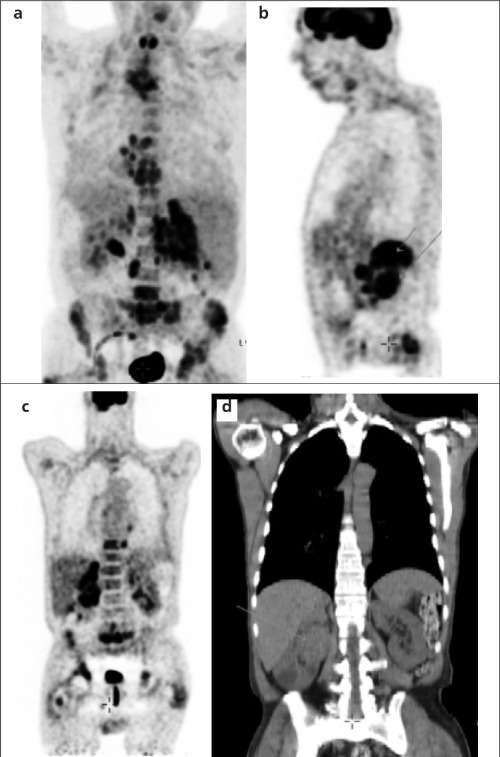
F-18 FDG PET/CT images showing thoracic and abdominalmultiple para-aortic hypermetabolic lymph nodes, increased F-18 FDGuptake in multiple foci of thoracic and lumbar vertebrae, and in thepelvic bones with osteolytic lesions showing up as patches on CT(a: MIP image; b: sagittal section; c: coronal section and d: coronal CTsection). There was clearly a pathologic uptake of the soft tissuelesion originating from the right kidney measuring 6x5x3 cm andbilaterally in the surrenal glands (left thickened surrenal gland measuring2x1x1 cm, and right surrenal gland measuring 12x9x4 cm)
